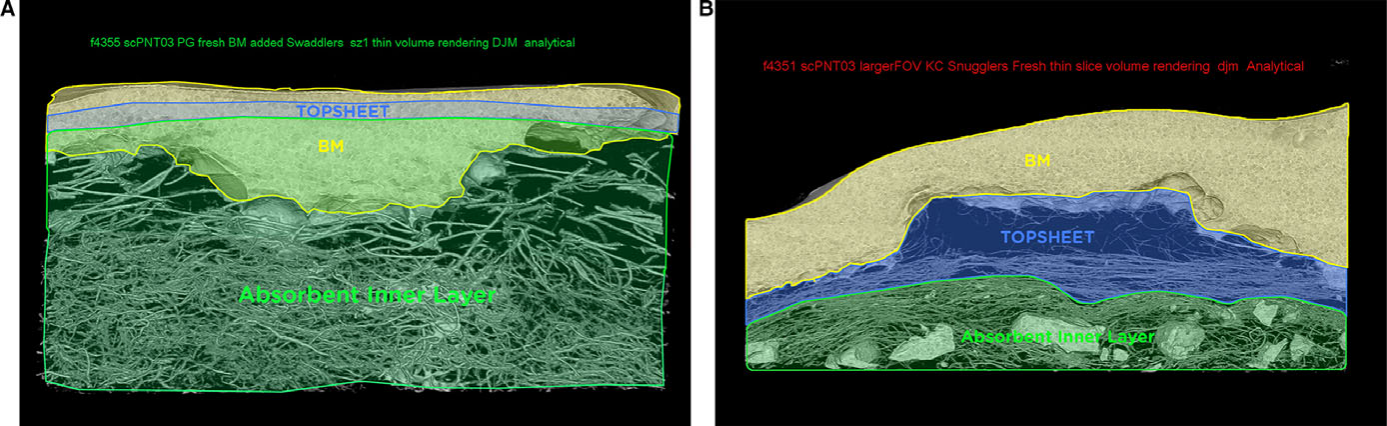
CT images of stool penetration below topsheet into absorbent core. A, Meshlike apertured diaper. B, Nonapertured diaper
